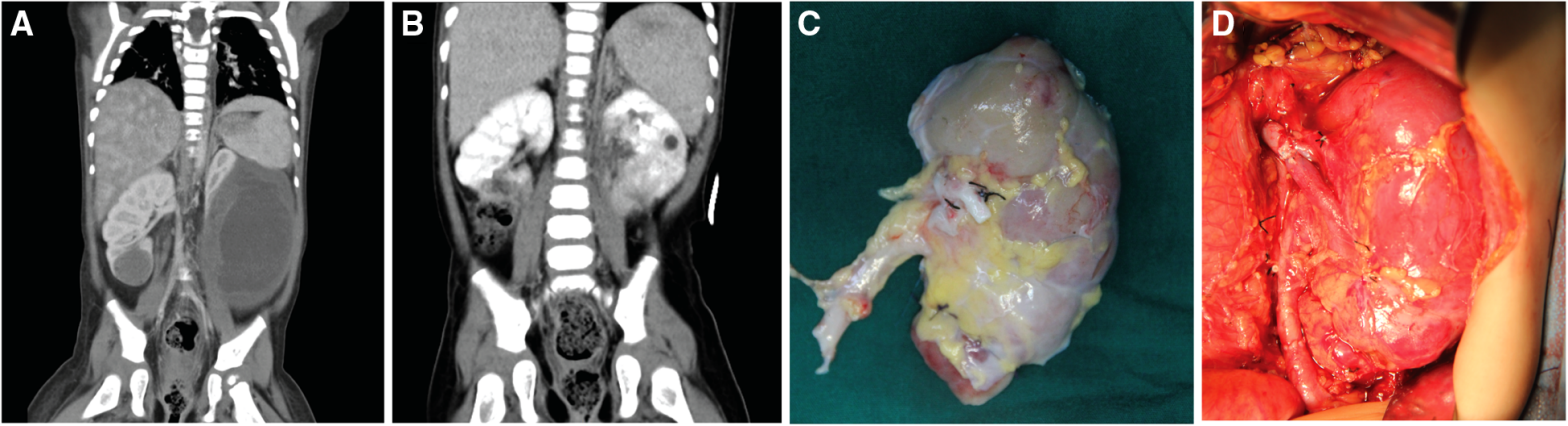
Preoperative images of patients and photos of the surgical area. (A) The preoperative enhanced CT image of patient No. 8 showed a tumor size of 83 × 62 × 116 mm in the lower pole of the left kidney and a tumor size of 28 × 23 × 26 mm in the lower pole of the right kidney; (B) The post-operative enhanced CT image of patient No. 8, the renal tumor has been completely resected; (C) The left kidney was completely stripped of the tumor *in vitro*, and the residual kidney was sutured; (D) The left kidney was autotransplanted.
